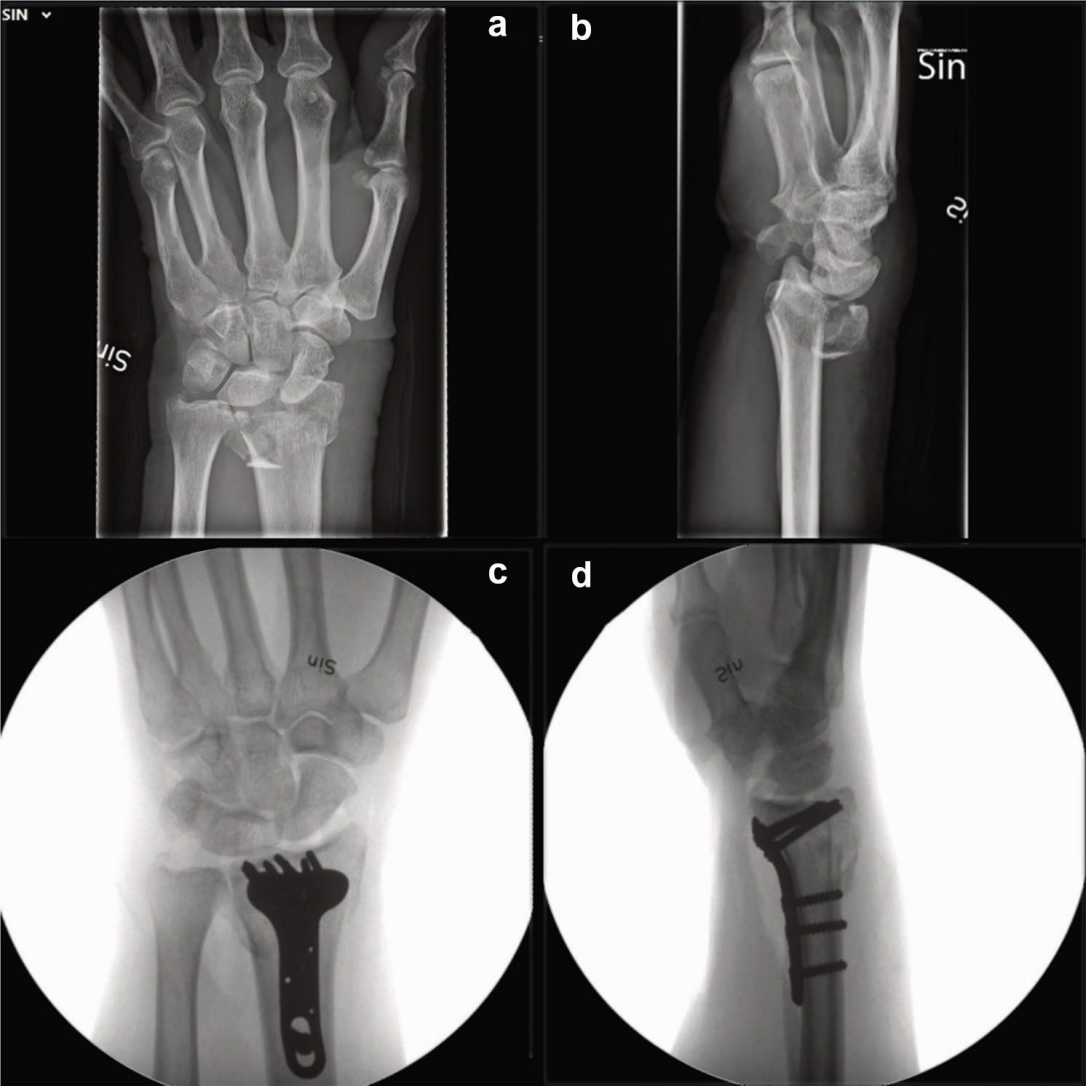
Pre- and intraoperative radiographs. Patient number 6
